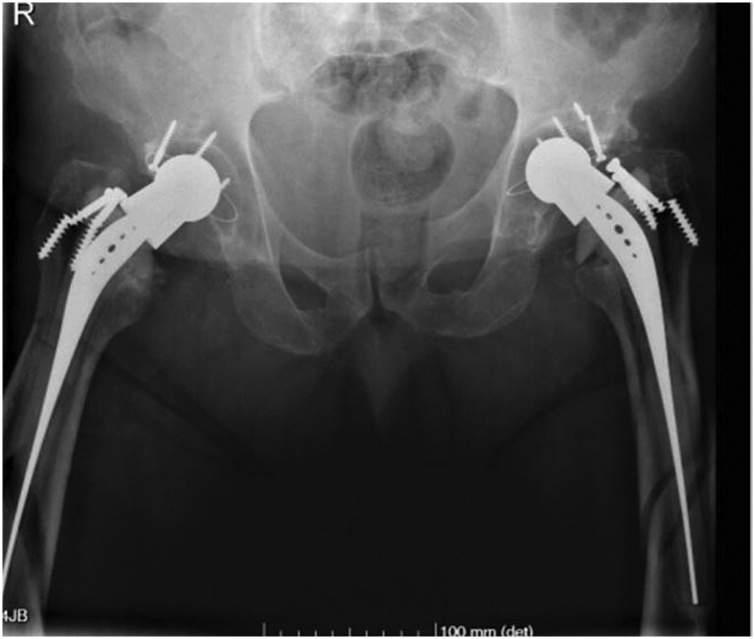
Preoperative anteroposterior radiograph of the pelvis showing bilateral Vancouver type-B2 periprosthetic femoral fracture.
